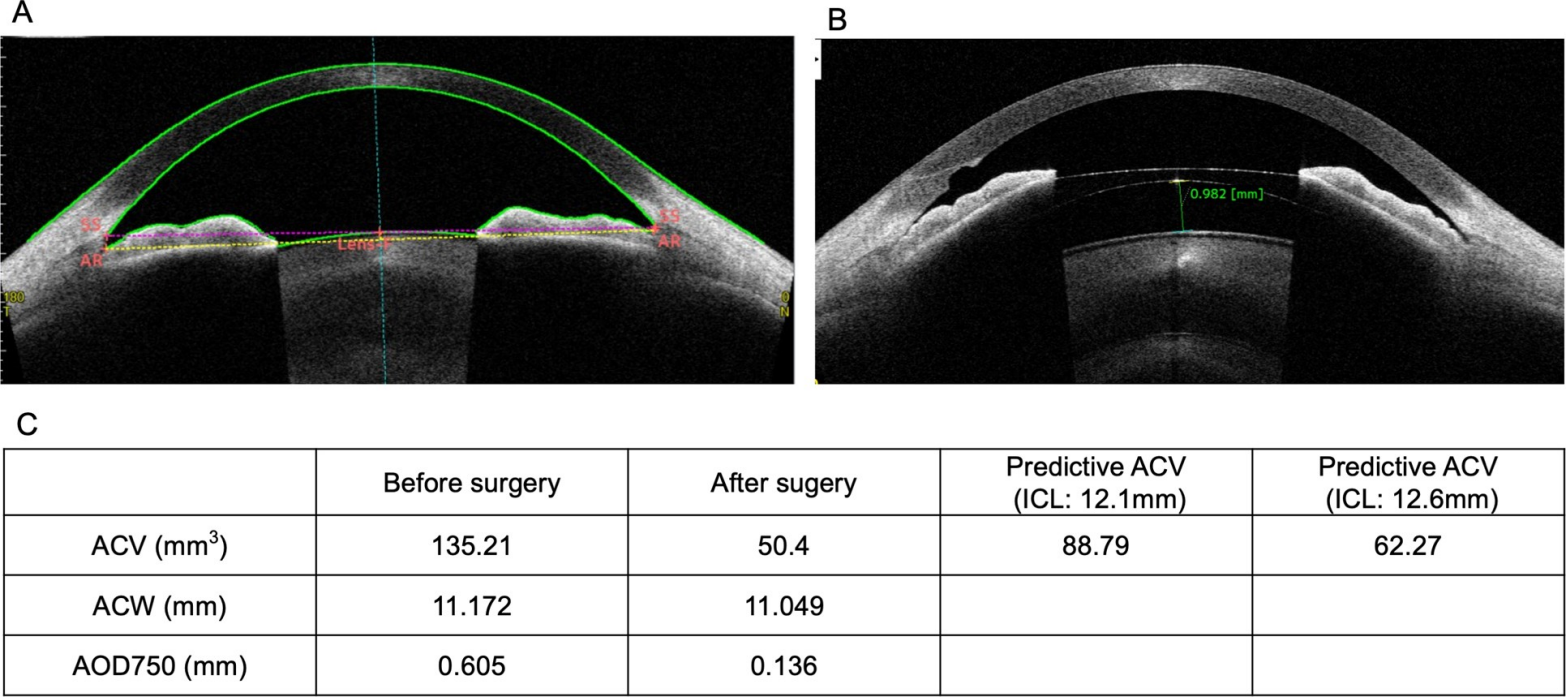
Case presentation showing narrow angle after implantable collamer lens (ICL) implantation. Anterior segment optical computed tomography images at the horizontal meridian before and after ICL implantation are shown (A, before; B, 3 months after surgery). In this case, the ICL size (12.6 mm) was calculated using the vault calculation equation, and a 12.6-mm ICL was implanted. As a result, angle narrowing occurred after surgery. The predictive postoperative ACV value (C) after the 12.6-mm ICL implantation was apparently low, whereas implantation of the 12.1-mm ICL was more suitable according to the postoperative ACV prediction. The generated ACV prediction equation may help determine the appropriate ICL size for such cases.
